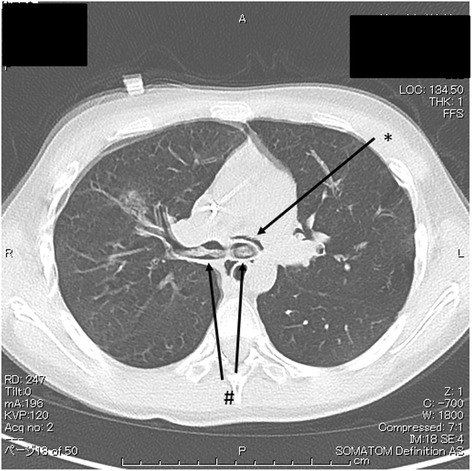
A chest CT before ICU admission. The arrows show a large high-density lesion in the carina over the bilateral bronchi (#) and mediastinal emphysema (*)
